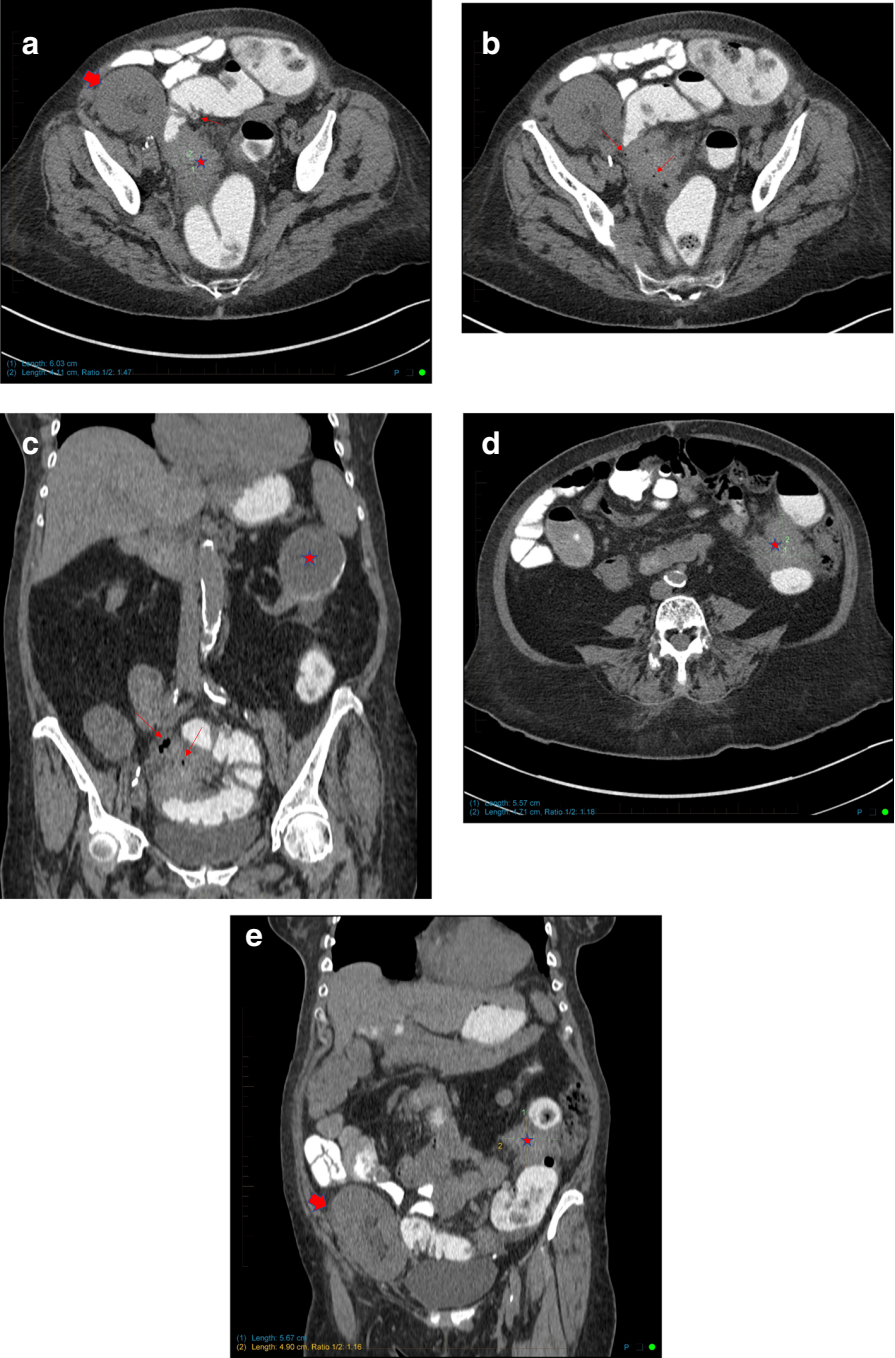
Abdomino-pelvic CT scan images. a Axial image showing a 6x4cm non stenotic mass (red star) adjacent to the distal sigmoid in a diverticular segment (thin red arrow). The transplanted kidney is partially seen in the right lower quadrant (thick red arrow). b and c Axial and coronal images showing small bubbles of extra-luminal air trapped in the mesosigmoid mass (red arrows). Incidentally, a partially calcified renal lesion is seen in the left native kidney (red star). d and e Axial and coronal images showing a second non stenotic mesocolic mass adjacent to the descending colon
